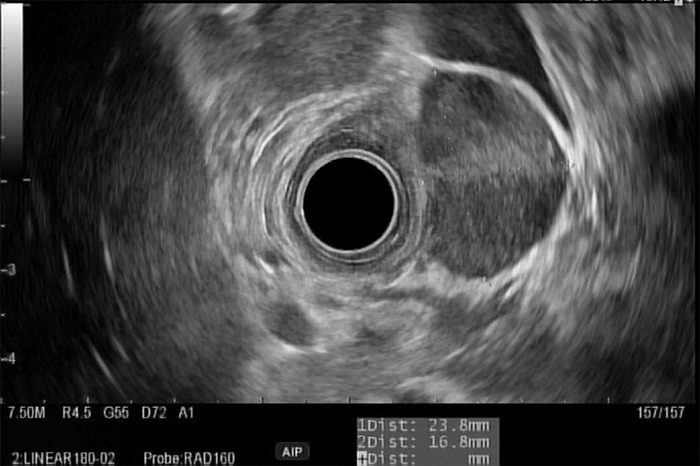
Endoscopic ultrasound in duodenal bulb showed a 23.8 mm × 16.8 mm submucosal, hypoechoic mass in the muscularis propria.
